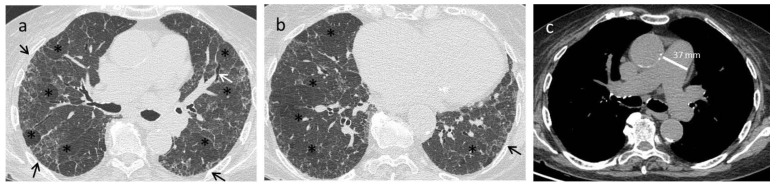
Pulmonary Hypertension. An 82-year-old female with a diagnosis of SSc. Axial High Resolution CT images show extensive subpleural reticulations (black arrows) and traction bronchiectasis/bronchiolectasis (white arrows), compatible with a UIP probable pattern (a,b). Signs of pulmonary hypertension, a common complication of this disease, can also be appreciated. On high resolution CT scan there is diffuse mosaic attenuation (*) with enlarged peripheral vessels (a,b), and a dilatation of the main pulmonary artery can be recognized using the mediastinal window setting (c).
